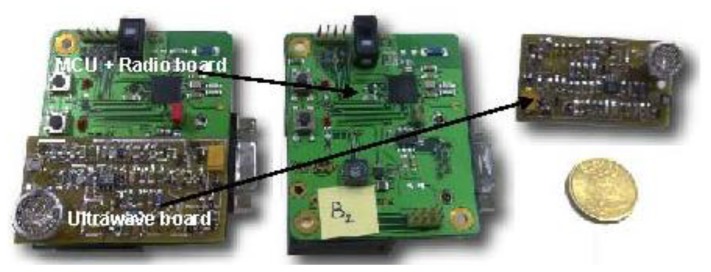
RF and ultrasonic devices.
